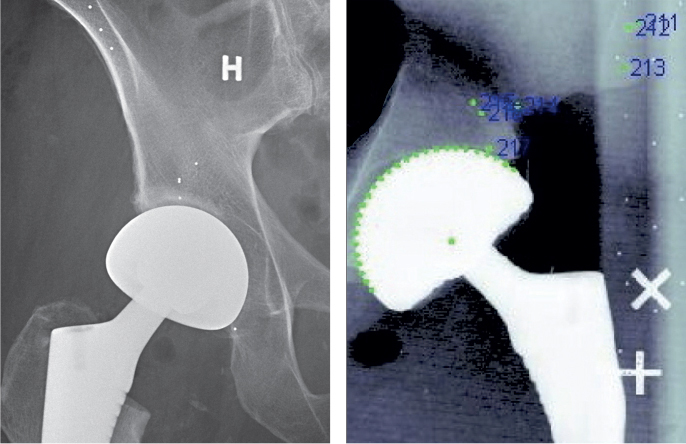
The image on the left is a conventional radiograph showing a bipolar hemiarthroplasty of the right hip. Tantalum markers have been implanted around the acetabulum, in the anterior superior iliac spine, and in the superior pubic ramus. To the right is a radiostereometric image with tantalum markers in the pelvis (numbered) and tantalum markers in the calibration cage. The center of the outer prosthetic head has been computer-calculated using edge detection.
